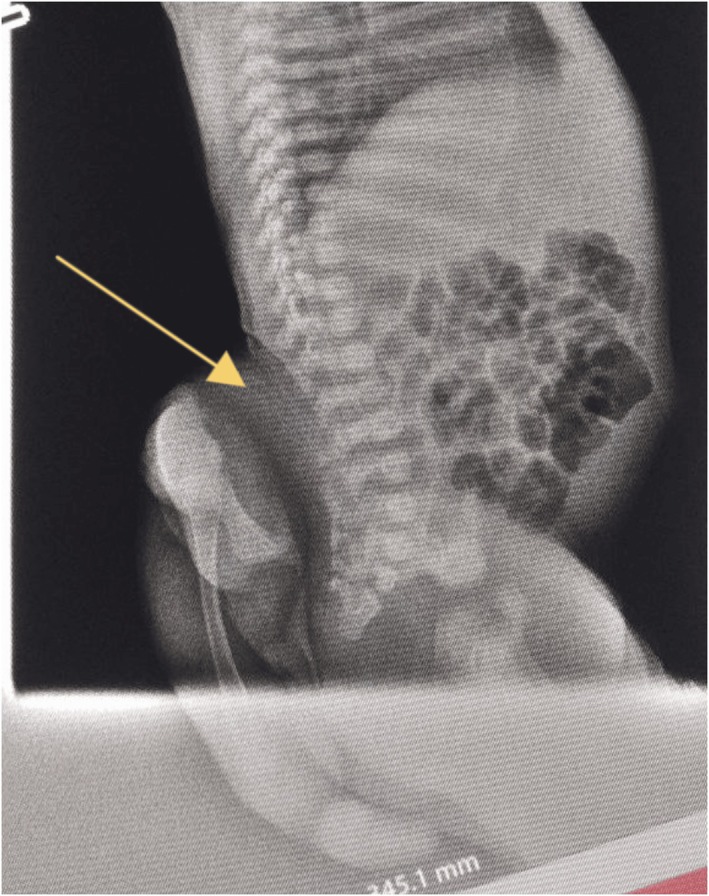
Plain lateral radiograph demonstrating posterior soft tissue mass. Lateral plain radiograph of the lumbosacral spine in a 4‐day‐old female neonate with rachipagus parasitic twinning. The yellow arrow indicates the posterior soft tissue mass containing rudimentary, partially formed osseous elements attached to the site of vertebral dysraphism.
